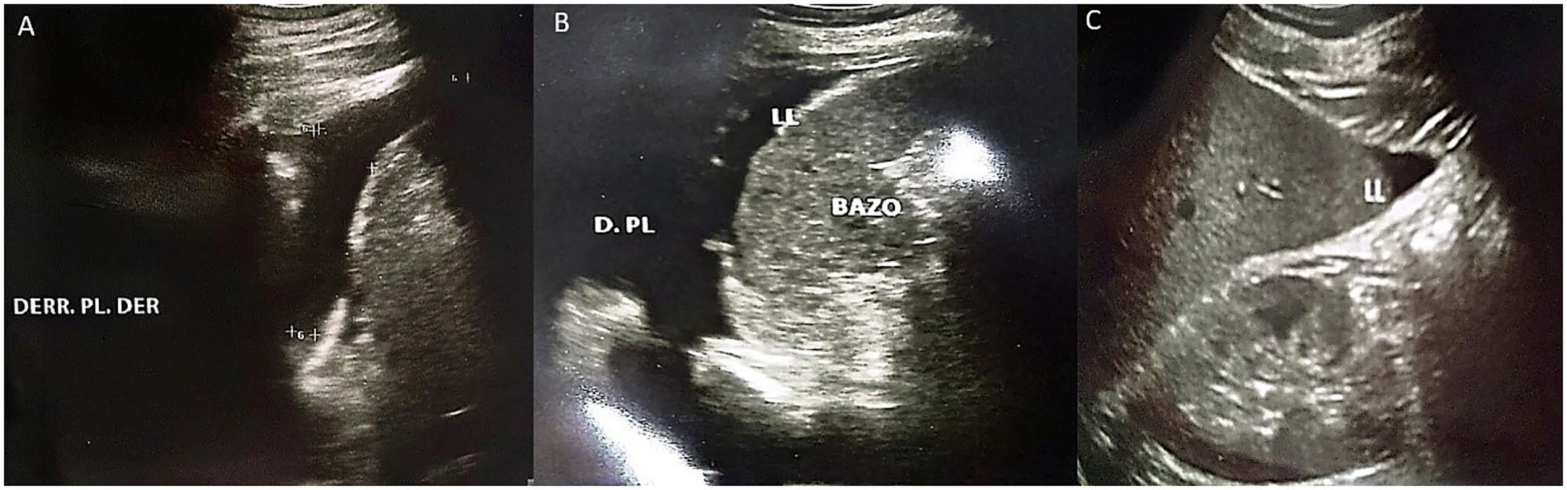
Abdominal ultrasound performed on the third day of hospitalization. (A) Presence of right pleural effusion is evident. (B) Presence of left pleural effusion is evident, as well as free fluid bordering the spleen. (C) Presence of free fluid bordering the liver.
